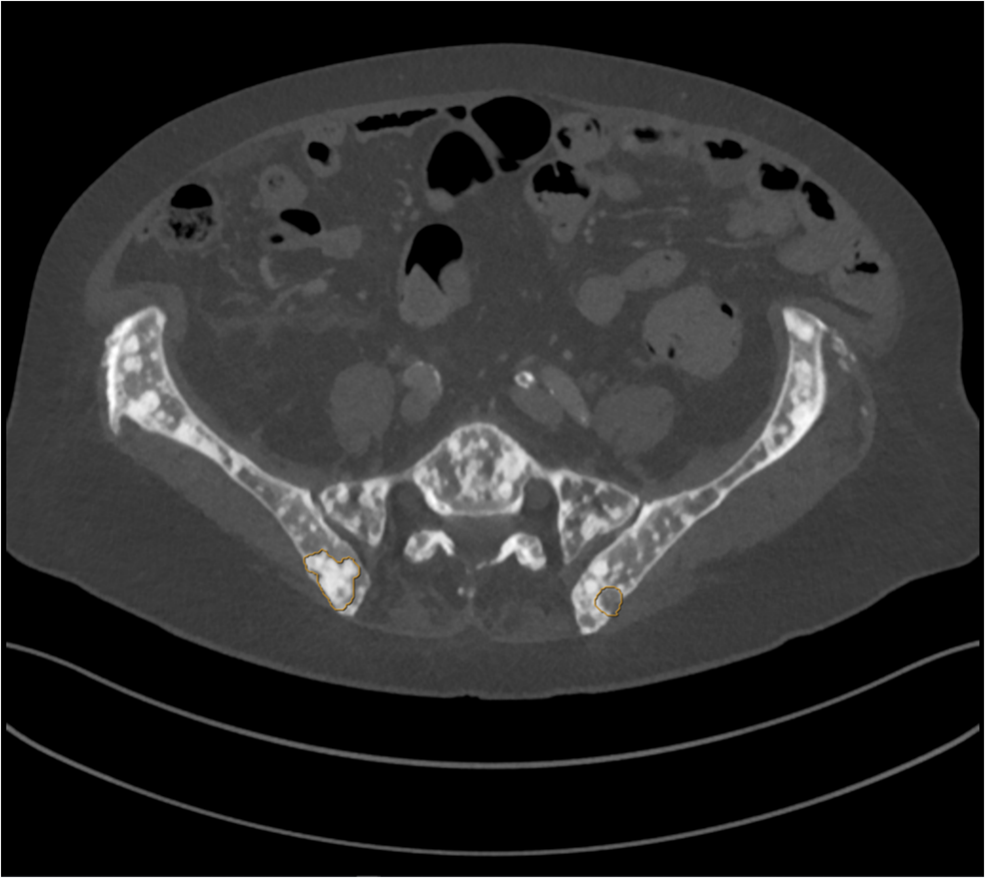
Example image from the DROID skeletal radiology dataset. Axial CT slice with two segmented lesions. On the right side of the patient, one sclerotic lesion is segmented. On the left side of the patient, one lytic lesion is segmented. Observe that the whole pelvic bone is disseminated by both sclerotic and lytic bone metastases
